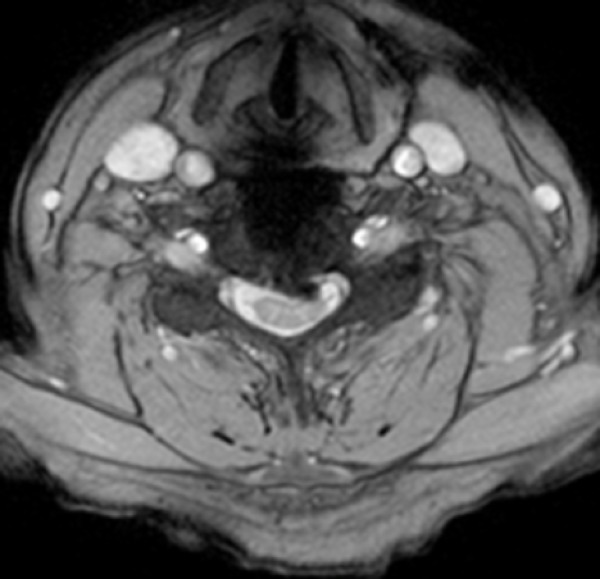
(Case 2) Preoperative axial MRI of the cervical spine
